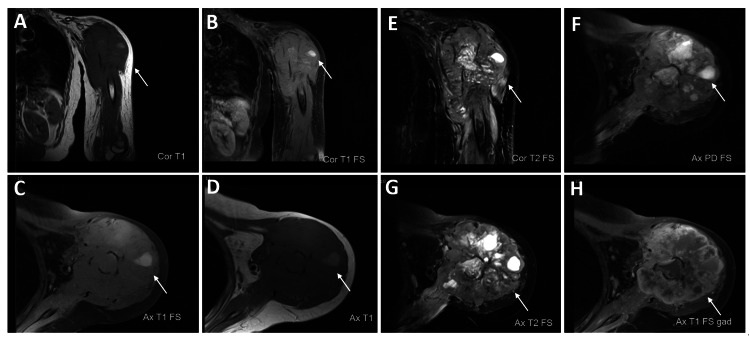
Further characterization of left humeral aggressive bony lesion A-H: The known mass lesion about the proximal humerus is again demonstrated. It extends to involve the humeral head and extends inferiorly to the level of the mid-humeral shaft. This measures approximately 10.3 cm anterior-posterior by 8.3 cm medial-lateral by 15.9 cm craniocaudad. This replaces the marrow about the proximal humerus and there is associated soft tissue extension and pathologic fracturing. The lesion is predominately hypointense/isointense on T1-weighted sequences with some T1 hyperintense signal of the lateral deltoid region (A-D). The lesion is heterogeneous on fluid-sensitive sequences and there is an avid heterogeneous enhancement (E-H). This is in keeping with the known leiomyosarcoma.
